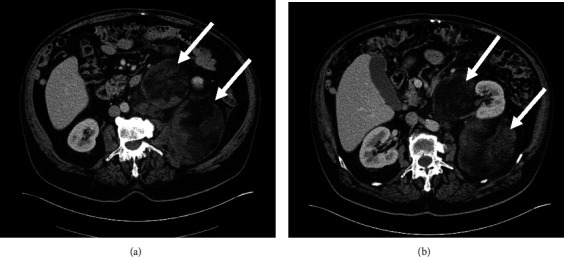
CT scan with multiple retroperitoneal myelolipomas (arrows).
